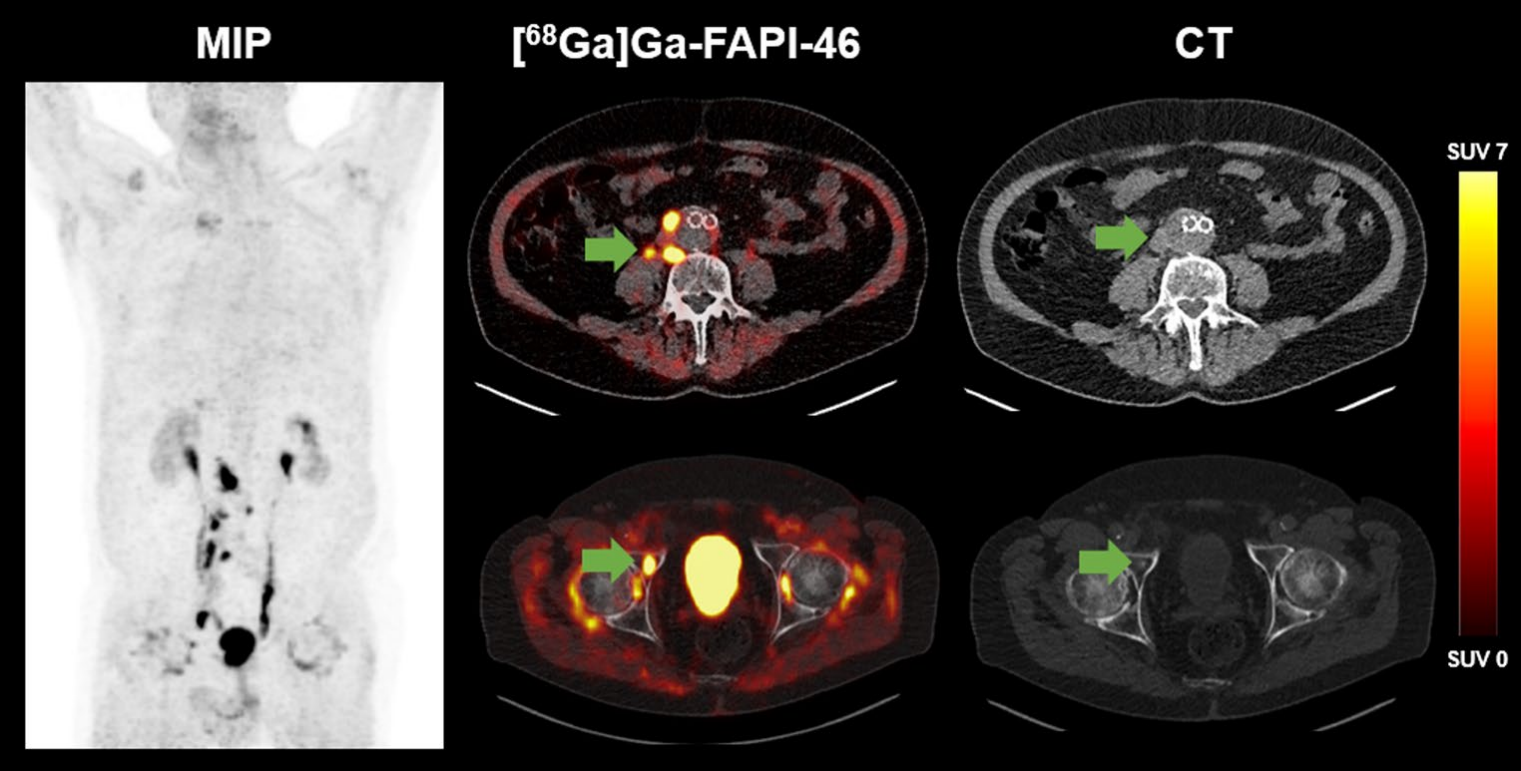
[^68^Ga]Ga-FAPI-46 PET/CT of an 81-year-old male patient with metastatic UC (LN, B). The arrows point to a paraaortic FAP-positive lymph node metastasis with a short axis diameter of 1.5 cm (SUV_max_ 45.4; upper row) and an osteoblastic lesion of the right acetabulum (SUV_max_ 18.7; lower row)
